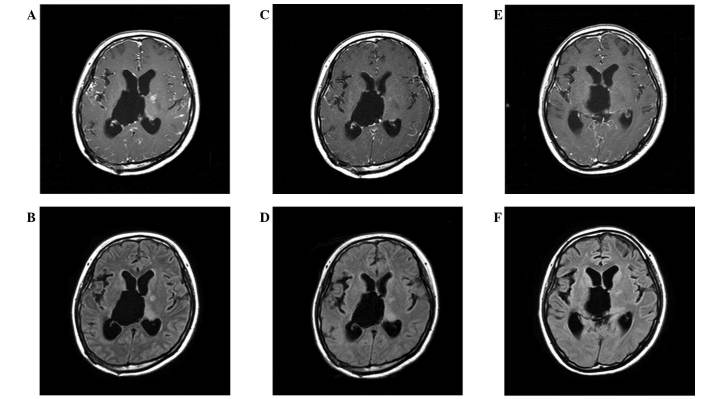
(A, B) MRI showed the progression of leptomeningeal dissemination following one cycle of TMZ and interferon-β therapy. (C, D) Leptomeningeal dissemination on MRI decreased following two cycles of TMZ and one cycle of bevacizumab from the beginning of recurrent therapy. (E, F) MRI showed no progression of leptomeningeal dissemination following three cycles each of TMZ and bevacizumab from the beginning of recurrent therapy. A, C and E: T1-weighted contrast-enhanced MRI; B, D and F: Fluid-attenuated inversion recovery imaging. MRI, magnetic resonance imaging; TMZ, temozolomide.
